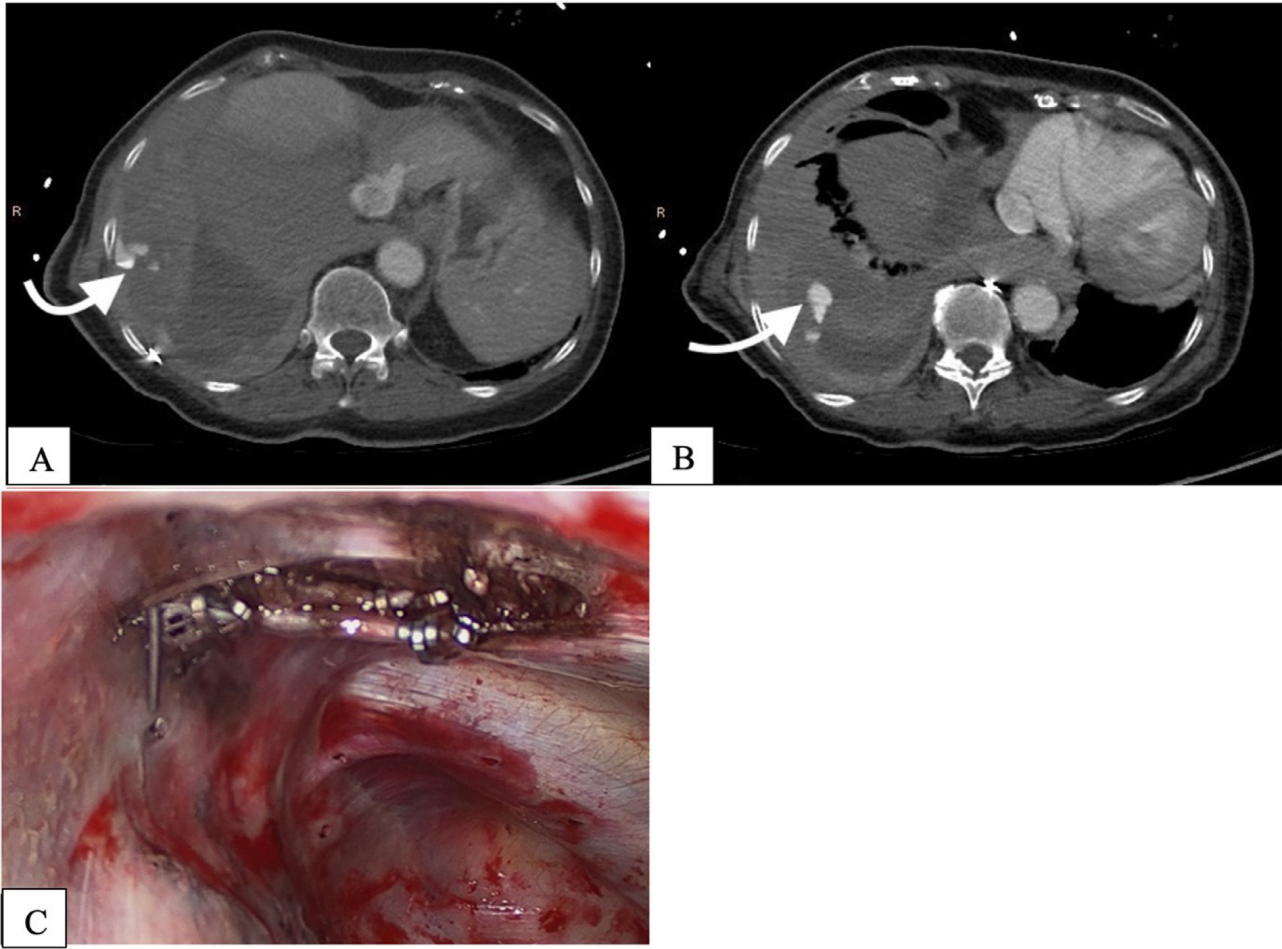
(A-C): A+B: Control CT-scan of the thorax shows persistent bleeding of 9th intercostal artery. C: Video-assisted thoracoscopy clipping of the intercostal artery.
